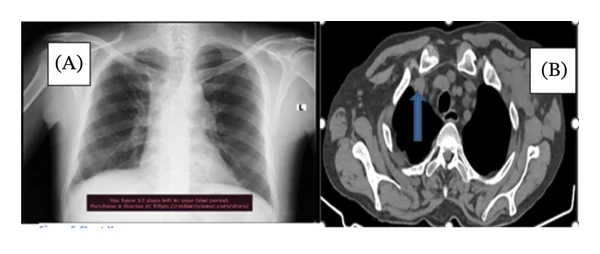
Chest imaging before and after immunosuppressive therapy: (A) a chest X‐ray with unremarkable findings before immunosuppressive therapy; (B) a noncontrast high‐resolution chest CT with multiplanar reconstruction on the mediastinal window, revealing mediastinal lymphadenopathy (blue arrows), highlighting radiologic evidence of pathology not apparent on plain radiography.
